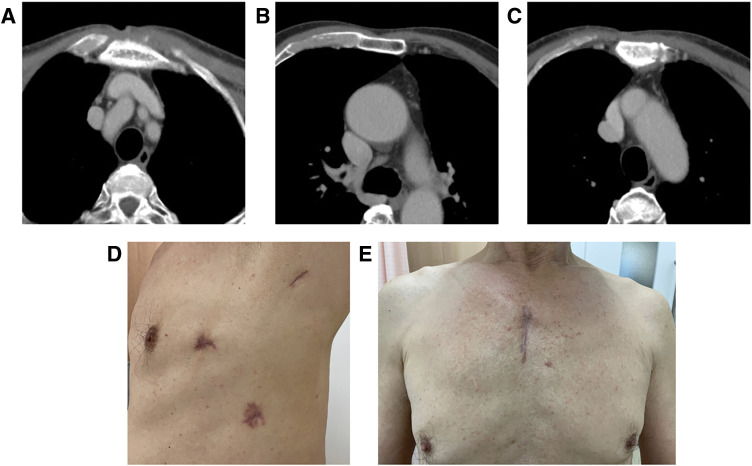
The CT scan, which was taken on postoperative day 135, showed improvement of the abscesses in the subcutaneous tissue of the anterior chest (A) and the anterior mediastinum (B). The destruction of the sternum was also improved (C). The surgical wounds on the lateral chest (D) and the anterior chest (E) are shown.
